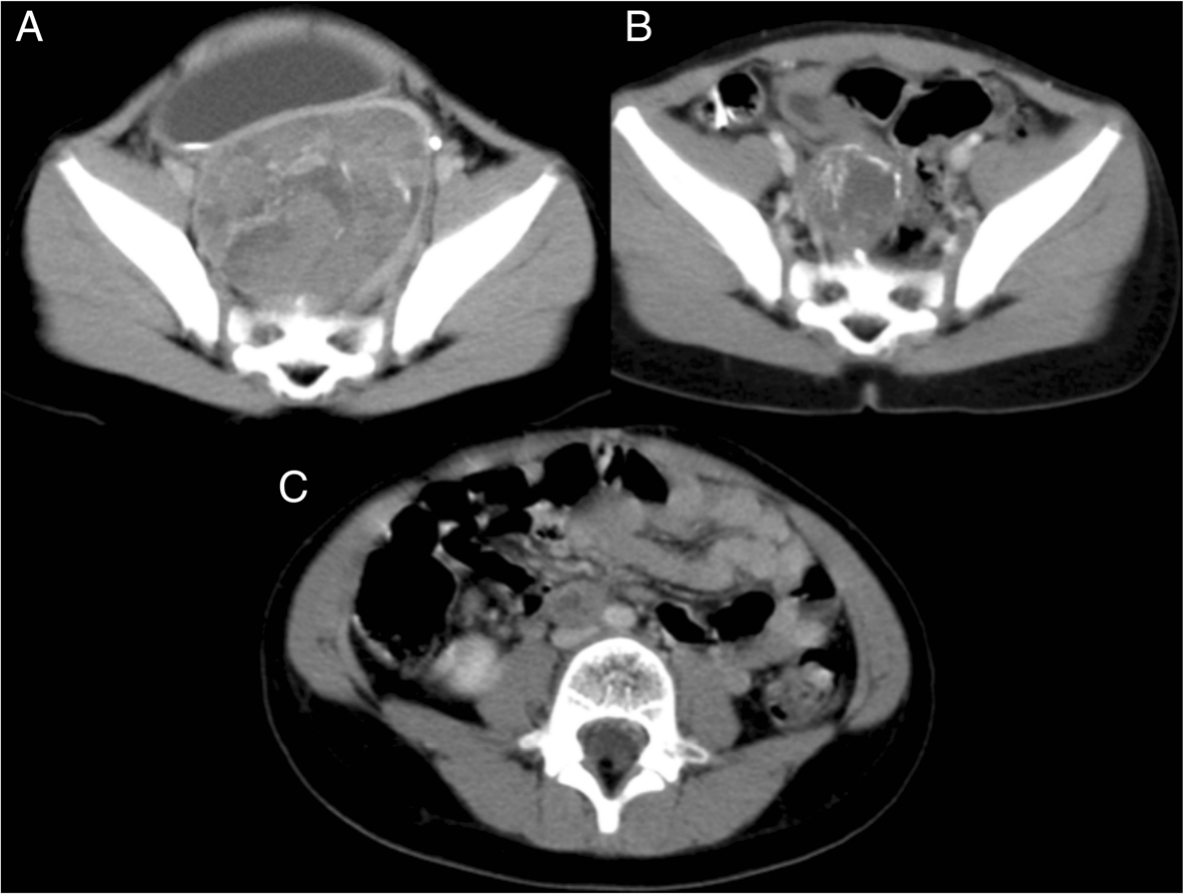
A: CT scans showing the tumor at diagnosis right hydronephrosis is also present; scans after 4 courses of CT, demonstrating partial response to therapy; C: recurrence of the tumor after six months from stop therapy. CT at diagnosis (A), after chemotherapy (B) and at relapse (C).
